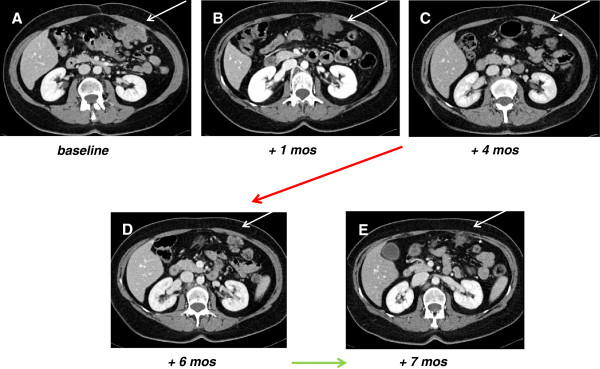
Computed Tomography scan (CT) tumor assessment. In panels A, B, C (white arrows) CT, venous phase, after contrast medium, shows a peritoneal metastasis at baseline, after 1 and 4 months of sunitinib with evidence of 30% decrease in tumor size and contrast uptake. Panel D shows an interval tumor progression at 6 months from baseline, i.e. after 2 months from sunitinib interruption, while panel E shows a new response at 7 months, 4 weeks after restoring sunitinib.
